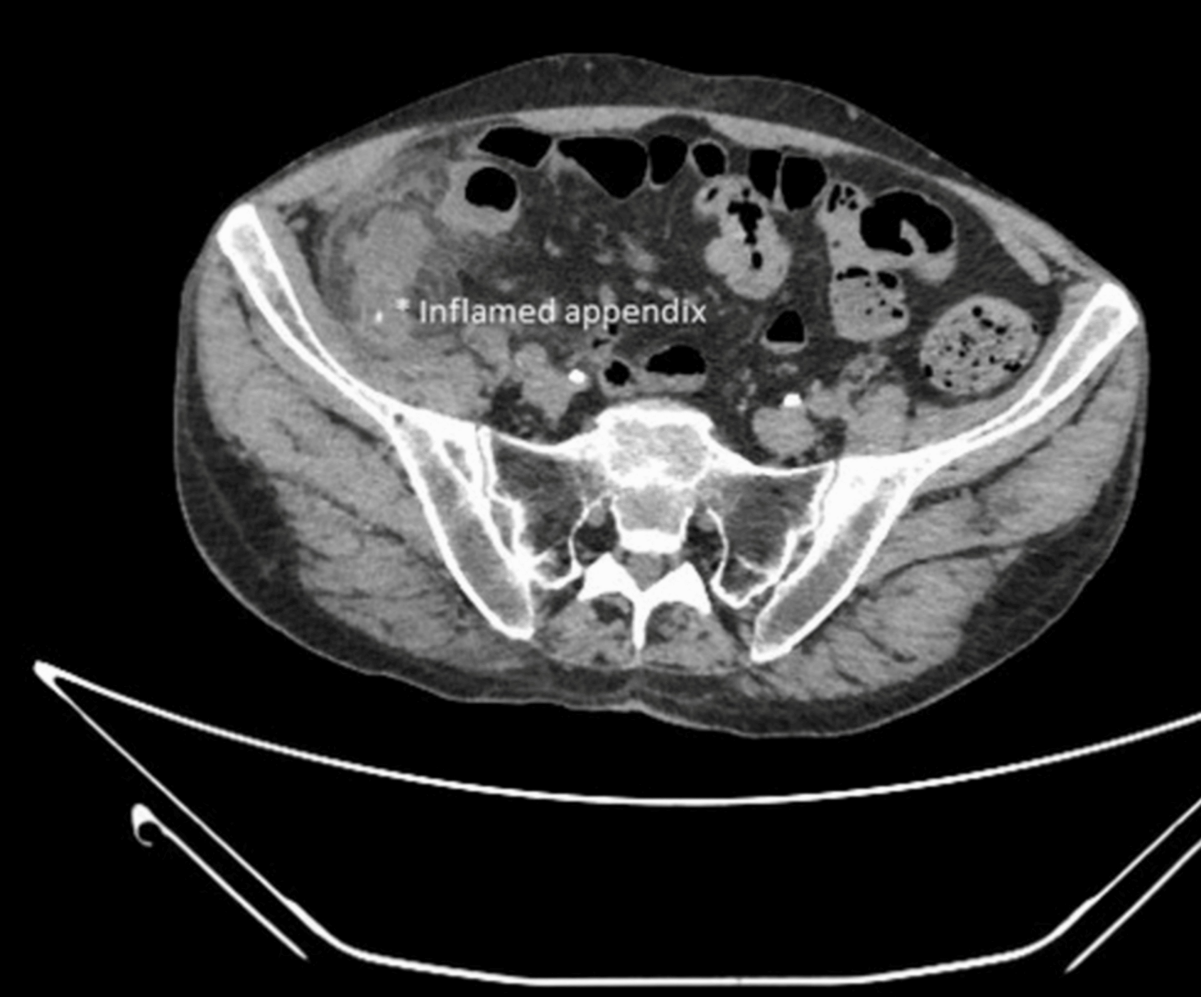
Acute appendicitis on CT scan The presence of pronounced dilatation (approximately 2 cm in diameter) and uneven thickening of the walls of the vermiform appendix indicates the presence of brief endoluminal calcific formations, likely attributed to coprolites.
